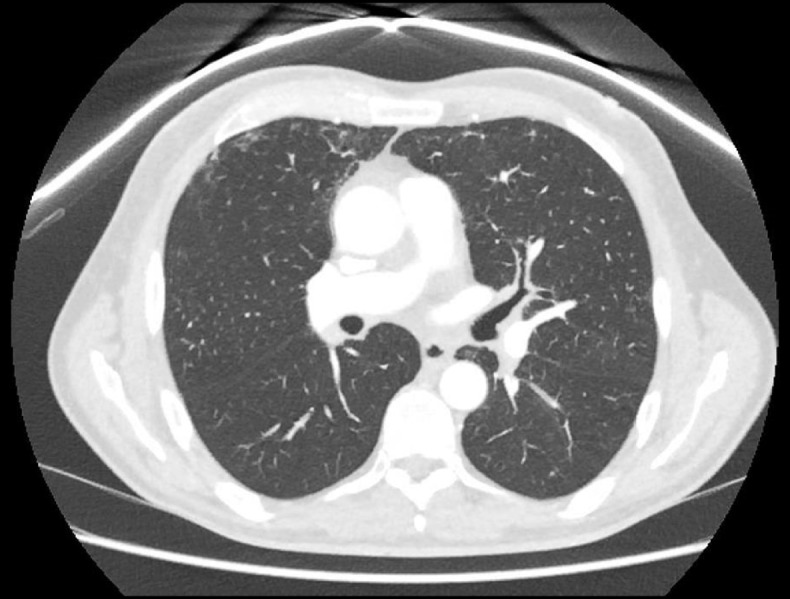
Slice from computed tomography imaging of the chest at re-presentation demonstrating bronchial wall thickening, in this instance particularly notable in the left upper lobe bronchus and proximal portion of the left anterior segment bronchus (Lb3)
